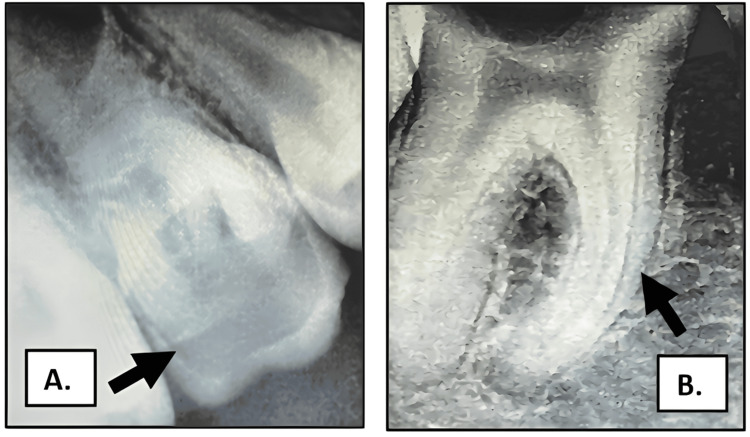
Preoperative radiographs showing deep occlusal caries in teeth 16 and 46 (A) Deep occlusal caries in tooth 16 (maxillary right first molar). (B) Deep occlusal caries in tooth 46 (mandibular right first molar).
